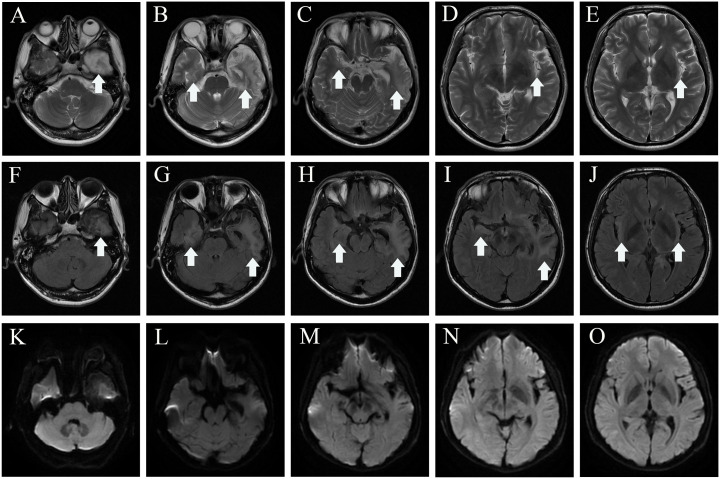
MRI of 2 months after discharge. Axial T2-weighted image (A–E), axial fluid attenuated inversion recovery sequence (F–J), and diffusion-weighted imaging (K–O) show the lesions in the left lobe, bilateral temporal lobe, bilateral insula lobe, and hippocampus were reduced, and no new-onset abnormal signal was found on DWI. The white arrowhead showing lesions in MRI. MRI, magnetic resonance imaging.
